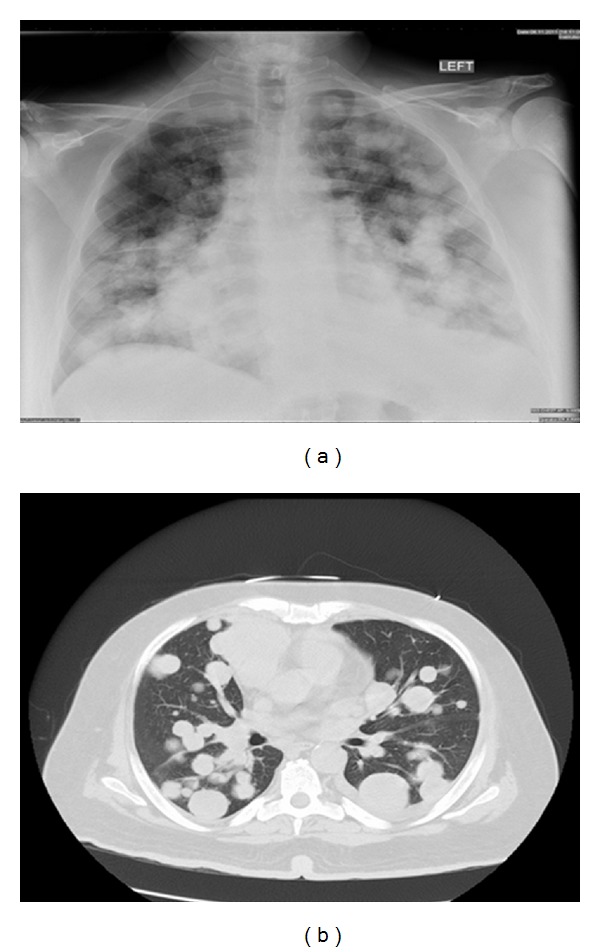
(a) Chest radiography showing multiple bilateral pulmonary nodules suggesting metastasis. (b) CT-scan of the chest revealing numerous pulmonary nodules suspicions for metastasis.
